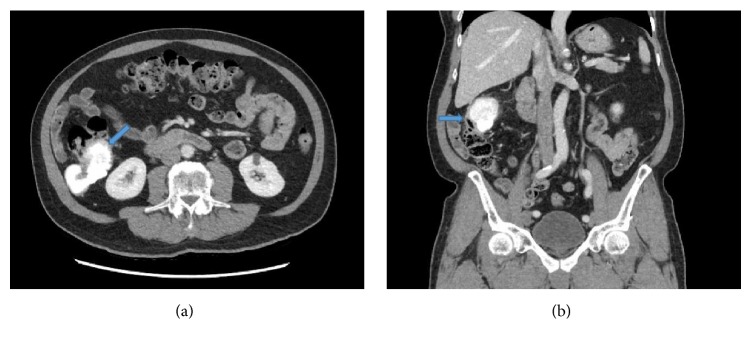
Contrast-enhanced CT scan images of right pericolonic mass: (a) axial and (b) coronal images demonstrate large partly exophytic lobulated mass (blue arrow) arising from the mid ascending colon with heterogeneously enhancement, consistent with mineralization or calcifications.
